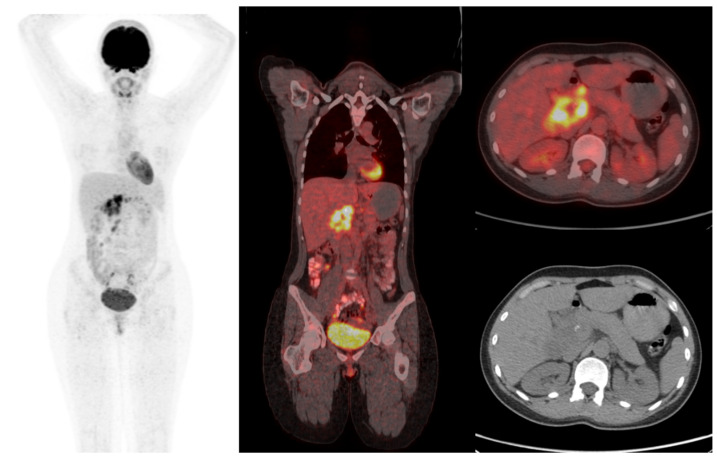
A 22-year-old previously healthy female evaluated for inflammation of unknown origin. Imaging by 18F-FDG PET/CT showed FDG uptake in the paraaortic, celiac, mesenteric, periportal, and perihepatic lymph nodes (SUVmax 15.4), raising suspicion for lymphoma. Subsequent biopsy confirmed *Mycobacterium tuberculosis* infection.
